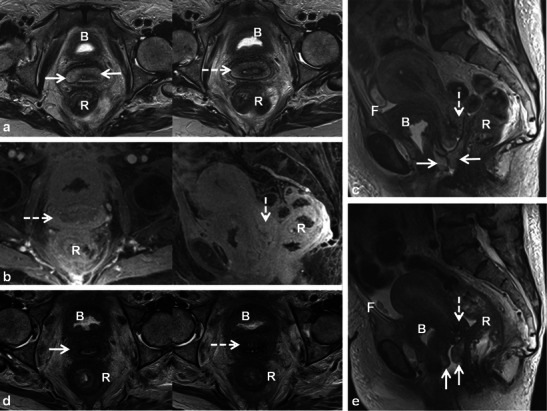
A 69-year-old woman with bladder adenocarcinoma status post transurethral resection receiving adjuvant pelvic external beam RT. Routine follow-up pelvic MRI demonstrates widening of the cervix and thickening of the anterior and posterior vaginal fornices with increased T2 signal intensity on axial and sagittal T2 TSE (*dashed and solid white arrows* respectively in a and c). Also note diffuse thickening and increased T2 signal in the rectum (*R*). Axial and sagittal T1 fat-saturated gradient echo images (c) after gadolinium injection also depict the findings (*dashed arrows*, *R*). Note pelvic free fluid (*F*), stranding and oedema in the pelvis. Follow-up pelvic MRI obtained 3 months later show similar but improving findings in the cervix (*dashed arrows*) and vagina (*solid arrows*) on axial (d) and sagittal (e) T2 TSE sequences consistent with acute radiation cervicitis and vaginitis. Note worsening radiation proctitis (*R*) with increasing pelvic free fluid (*F*). Bladder wall thickening and increased T2 signal (*B*) was felt to be due to radiation cystitis in this patient post resection, although residual or recurrent tumour could have a similar appearance
